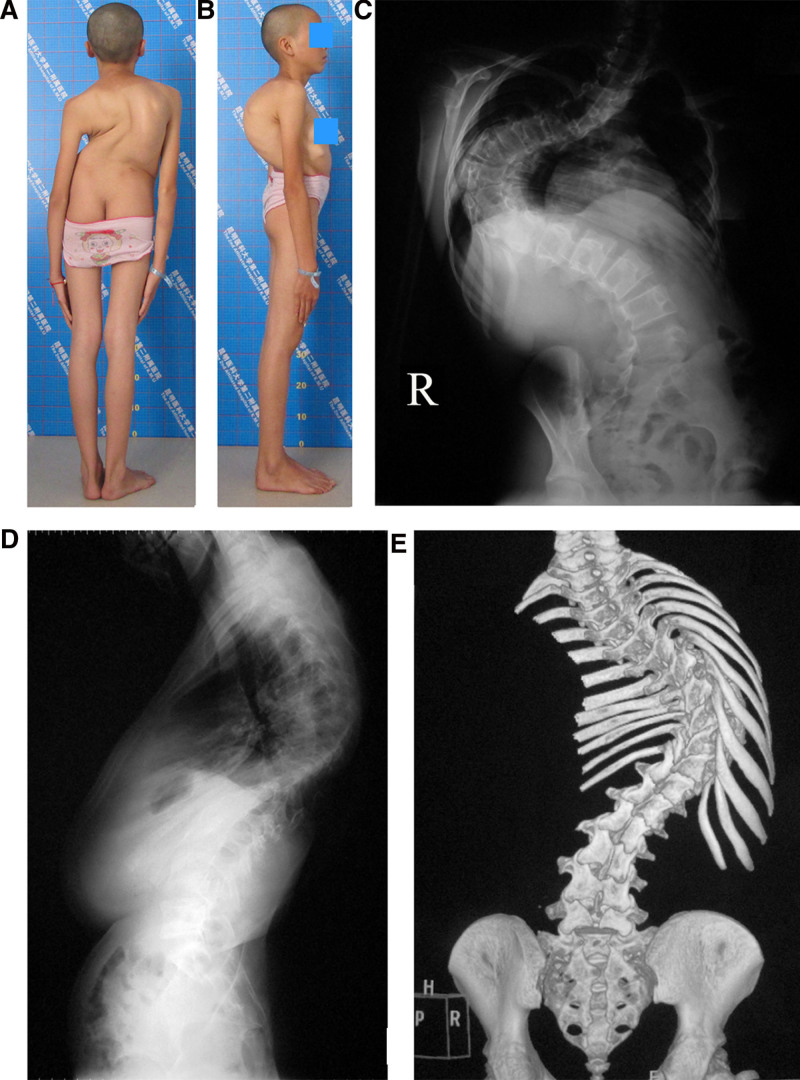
A 12-year-old female patient suffers from severe rigid scoliosis with trunk imbalance. (A) Preoperative deformity in the posterior view. (B) Lateral view. (C) A preoperative anteroposterior (AP) X-ray shows a Cobb angle of 132°, radiographic shoulder height (RSH) of 4.1 cm, C7-central sacral vertical line (C7-CSVL) of 3.2 cm. (D) On a lateral X-ray, the Cobb angle is 65°, and the sagittal vertical axis (SVA) is 4.0 cm. (E) Preoperative 3-dimensional CT image reconstruction showing a posterior view of the spinal deformity. AP = anteroposterior, C7-CSVL = C7-central sacral vertical line, CT = computed tomography, RSH = radiographic shoulder height, SVA = sagittal vertical axis.
